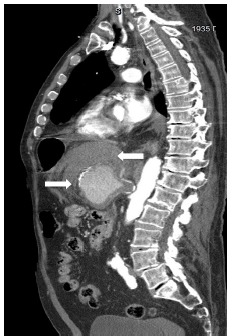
Computed tomographic angiography of the chest and abdomen demonstrating active extravasation of the celiac artery and splenic artery aneurysm (rightward arrow) with extensive stranding (leftward arrow), representing active hemorrhage.
